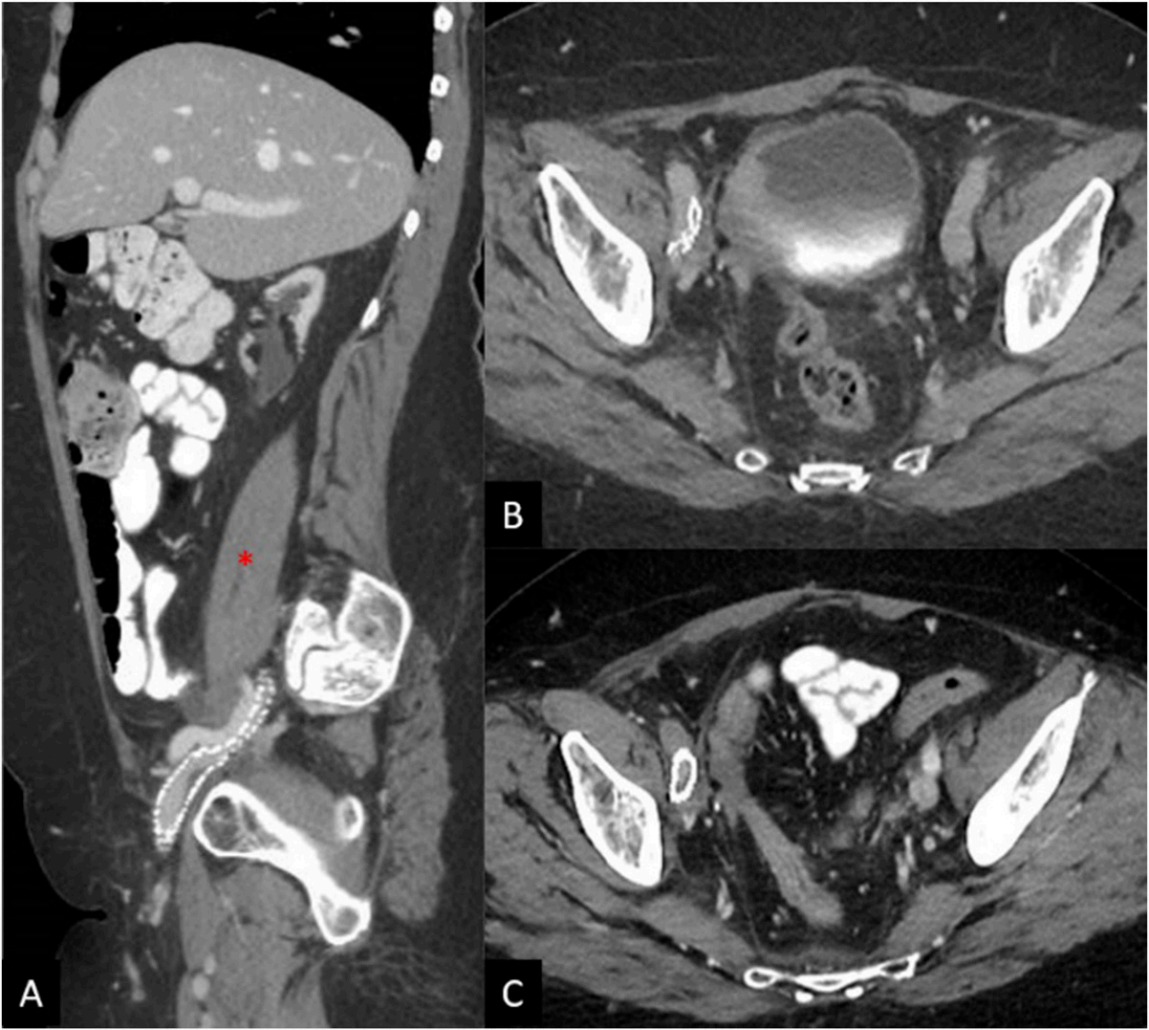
(A) Sagittal CT image shows compression of the stent in the external iliac vein. Pelvic tumor (*) is anterior to the right external iliac artery. (B) Axial CT image shows stent recoil with compression of the stent at the level of the right external iliac artery. (C) Axial CT image after balloon expandable stent placement in the right external iliac vein shows persistent, but improved stent compression. The right external iliac artery is now occluded with distal reconstitution of the common femoral artery (not shown).
